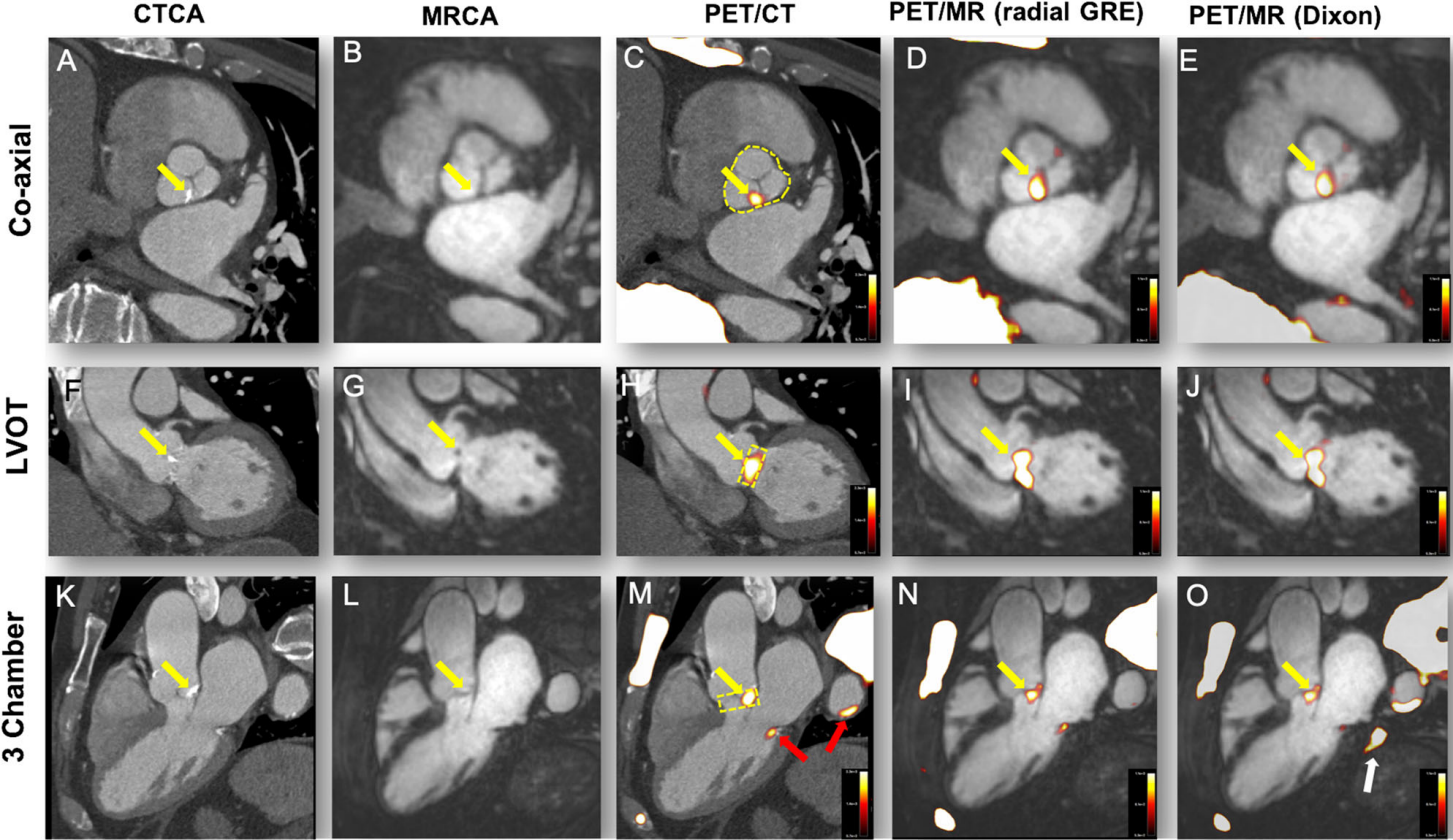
^18^F-Fluoride uptake in a patient with moderate aortic stenosis. The columns represent the imaging modality and rows the corresponding view. (A, F, G) Calcification of the aortic valve (non-coronary cusp predominantly, yellow arrows). (B, G, L) The coronary magnetic resonance angiogram in the same views. Calcification cannot be appreciated on MR but the raphe between the non-coronary cusp and left coronary cusp appears thickened (B). PET/CT shows uptake overlaying these areas of calcification (C, H, M). Note the uptake also over the calcified mitral annulus (M, red arrow) and arterial wall of the descending aorta (M, red arrow). Radial GRE-fused PET/MR shows ^18^F-fluoride uptake in the same areas as the PET/CT (D, I, N). (E, J, O) The corresponding views in the Dixon PET/MR attenuation correction map. Again ^18^F-Fluoride follows a similar pattern to PET/CT but note the image artifact in (O) (white arrow)
